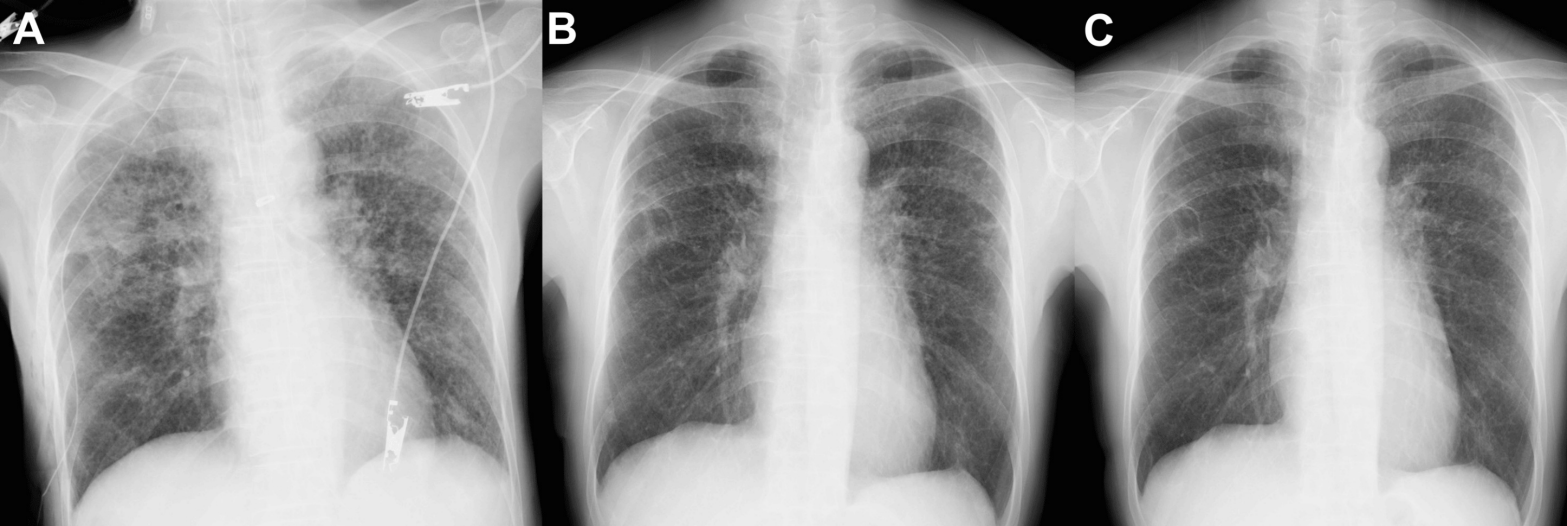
Follow-up chest radiographs Serial chest radiographs obtained immediately after surgery (A), two weeks after surgery (B), and three months after surgery (C) demonstrate gradual improvement of the diffuse reticulonodular opacities in both lungs.
